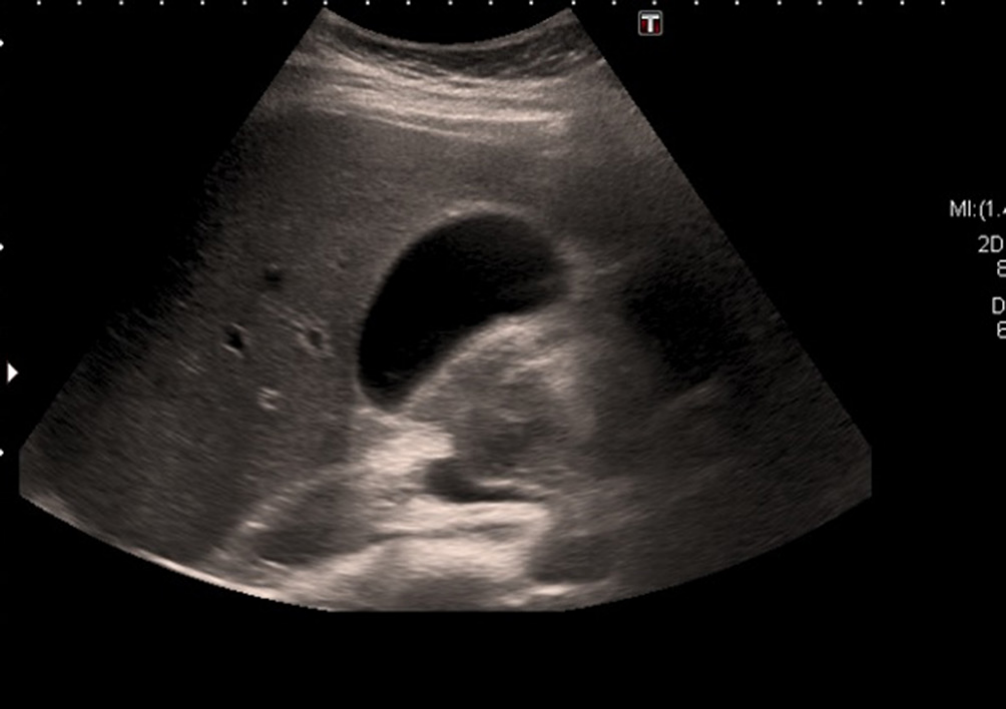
USS abdomen. The gallbladder looks entirely normal. There are no internal filling defects. Normal duct calibre. USS, ultrasound scan.
